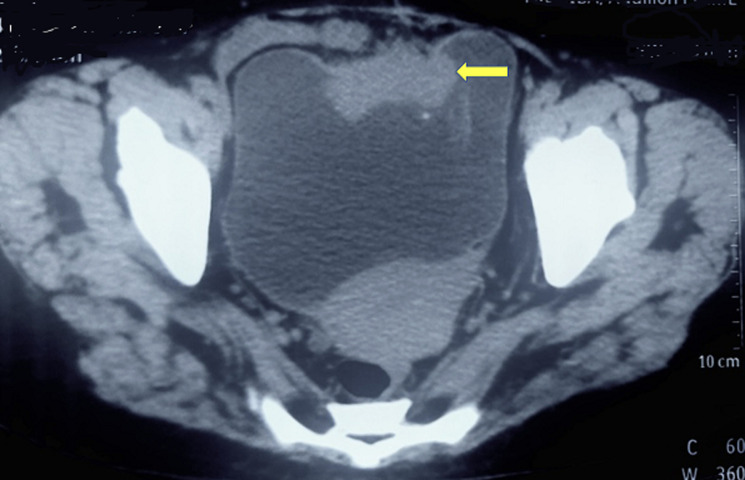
non-contrast computed tomography of kidney, ureter, and bladder region showing irregular, lobulated, enhancing soft tissue lesion along the anterior vesical wall measuring 2.1 x 4.7 x 4.8 cm with mild peri vesical fat stranding (arrow)
